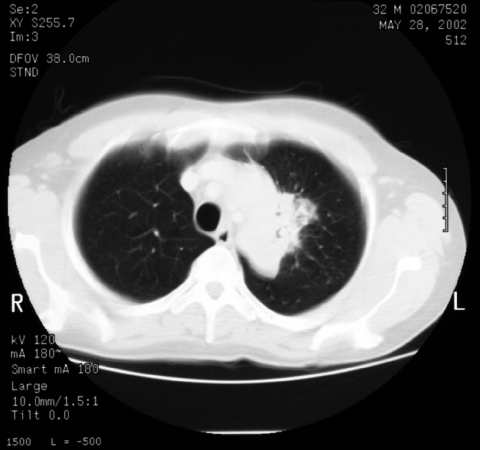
Computed tomographic scan of thorax at patient's initial visit. Results are highly suggestive of tumor.
